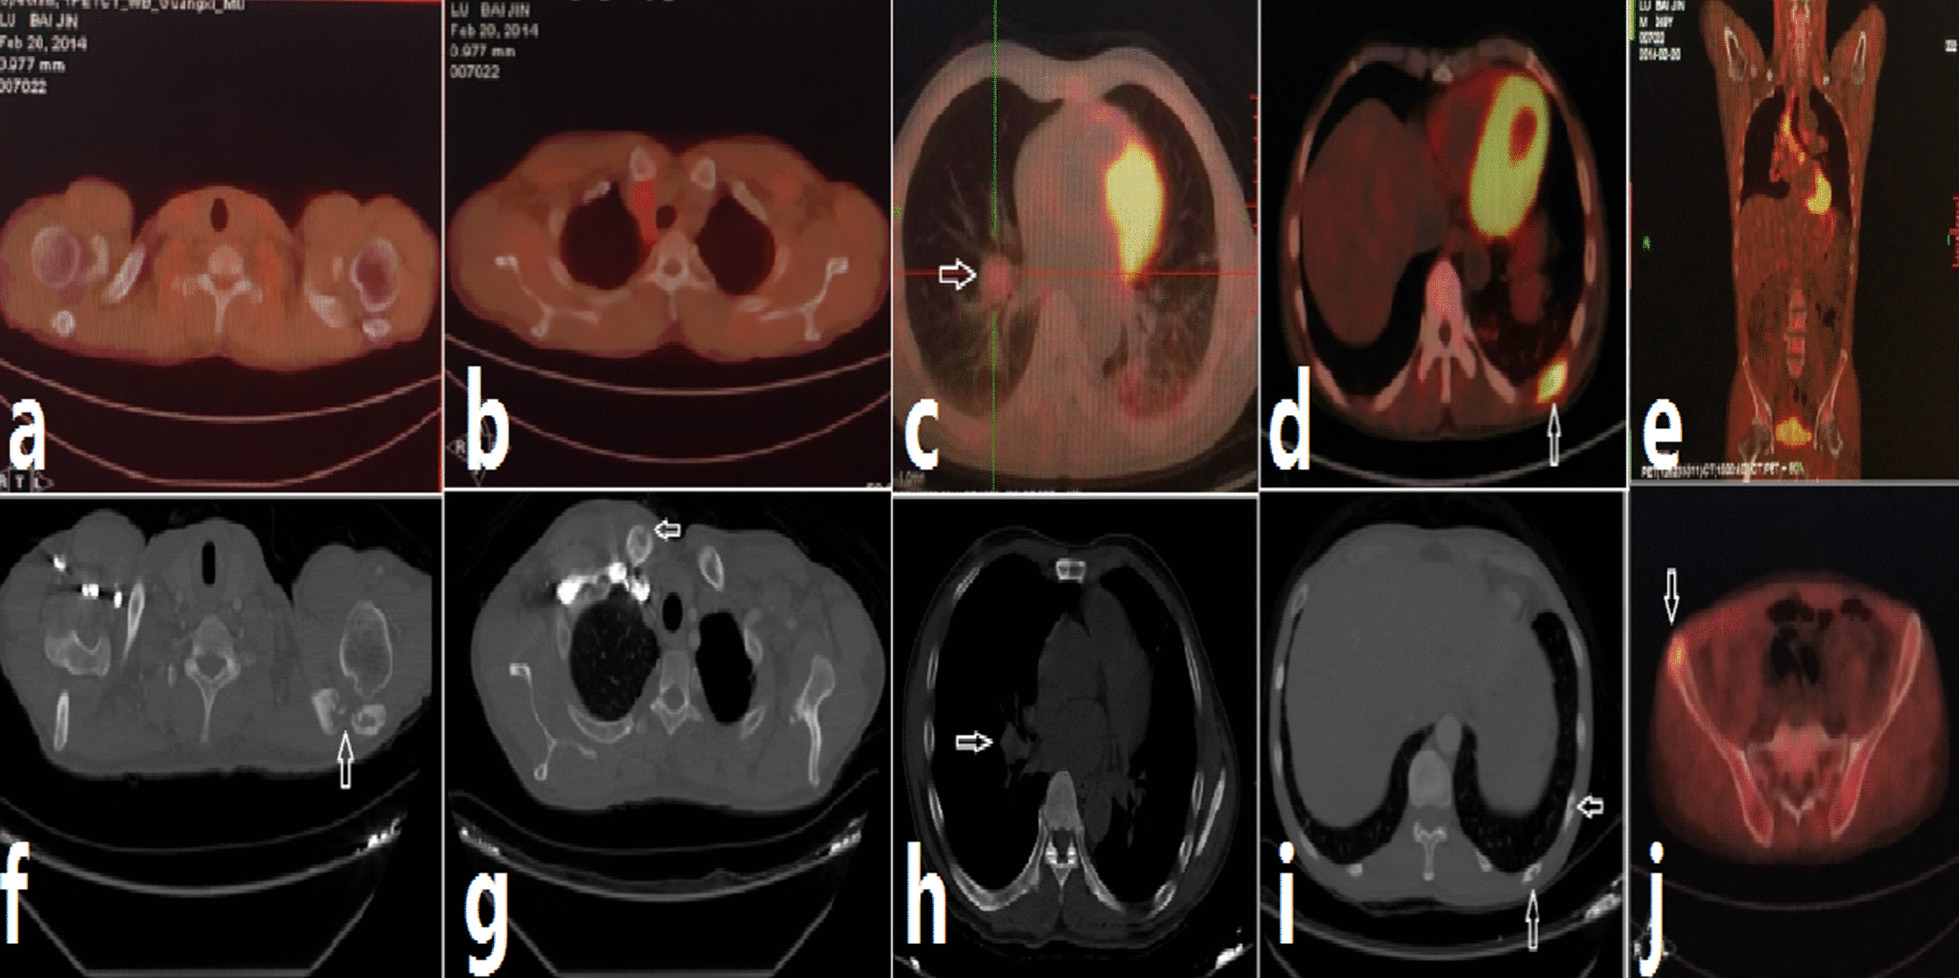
The comparison of imaging before and after receiving antifungal treatment. Positron emission tomography/computed tomography results showing the increase in metabolic activity in the right lower lung (c), mediastinal and hilar lymph nodes, ninth rib on the left (d), and right ilium (j). Standard uptake of 18F-fludeoxyglucose was 2.7, 8.0 (maximal), 6.5, and 4.2, respectively. After antifungal treatment (no chemotherapy), chest computed tomography showed well-defined aggravated osteolysis in the left acromion (f), clavicle (g), and ninth and tenth ribs on the left (i). Bone marrow cavities were also detected Panels (a), (b), (h) inside the image of Figure [1] are to compare the degree of osteolysis and lung infection in the same part of the patient before and after receiving antifungal treatment, so there is no redundant explanation. Panels (e) is a coronal view of PET/CT
